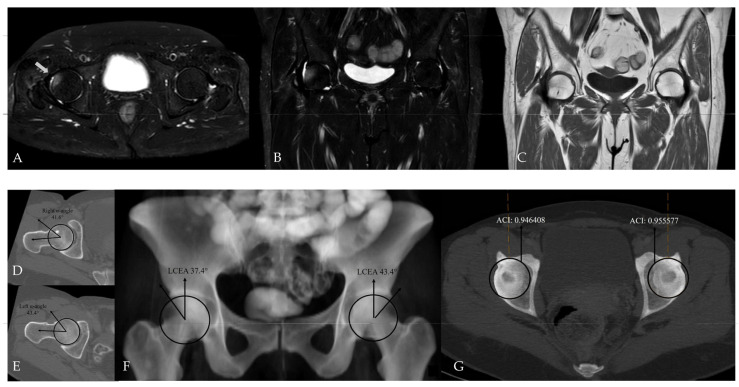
Hip MRI demonstrates focal bone marrow edema in the anterolateral aspect of the right femoral head on (A) axial (white arrow) and (B) coronal fat-suppressed T2-weighted images. (C) Coronal T1-weighted image shows no underlying structural abnormality. (D,E) CT demonstrates normal alpha angle measurements. (F) LCEA measurements indicate pincer-type femoroacetabular impingement only on the left side. (G) However, using an ACI cut-off value of 0.93, bilateral pincer-type femoroacetabular impingement can be demonstrated.
